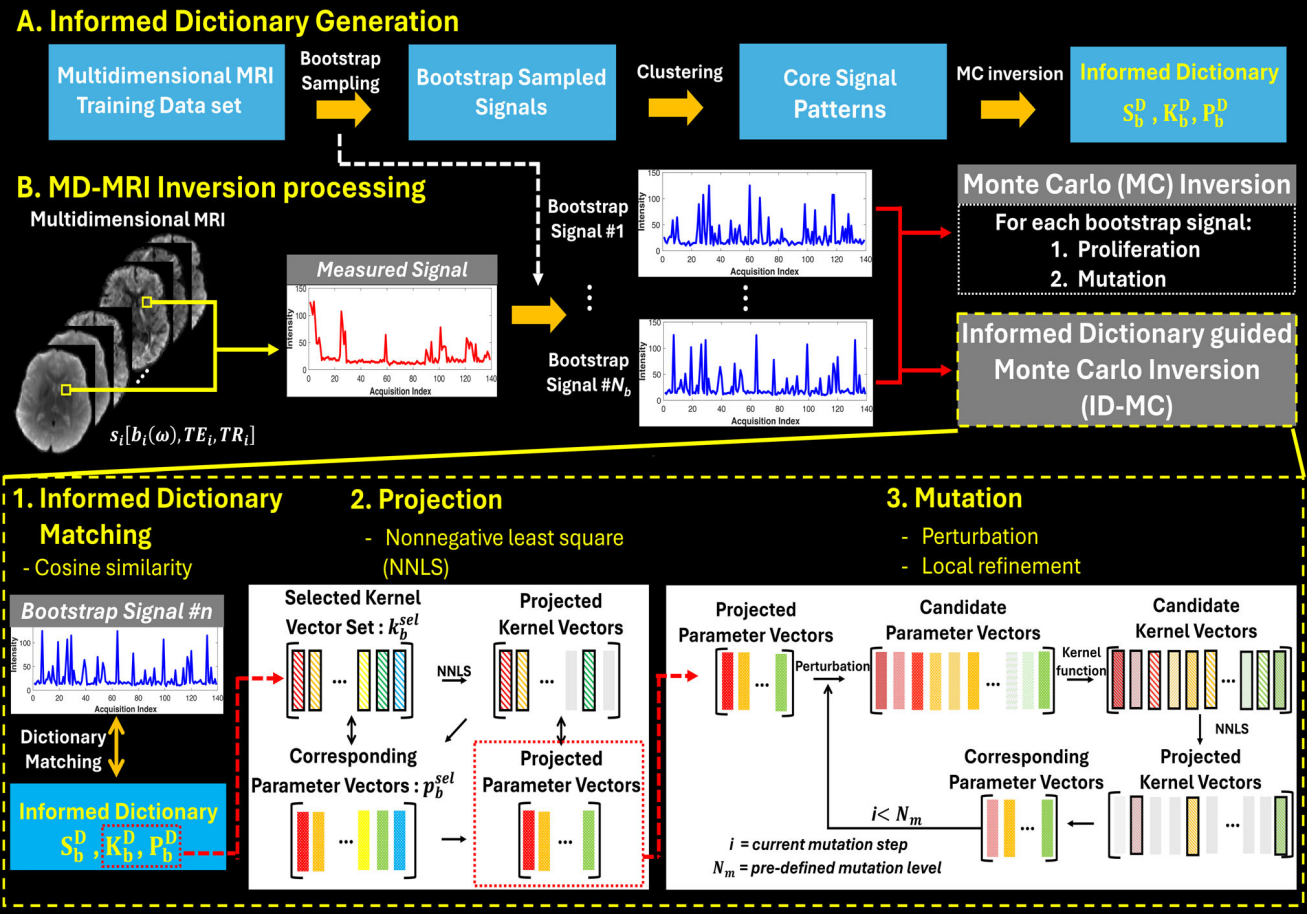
Overview of the Informed Dictionary‐guided Monte Carlo (ID‐MC) inversion framework for MD‐MRI. (A) Bootstrap‐sampled signals from training data are clustered to construct the informed dictionary, consisting of signal patterns ($S_{b}^{D}$), kernel sets ($K_{b}^{D}$), and corresponding parameter sets ($P_{b}^{D}$). (B) For each test bootstrap signal $s_{i}$, MC inversion consisting of proliferation and mutation steps is performed. Alternatively, the ID‐MC inversion performs matching with the informed dictionary, projects the signal onto the selected kernel space using nonnegative least squares (NNLS), and applies local refinement through parameter mutation. This process iteratively updates the kernel set and weights to estimate the accurate parameter set.
